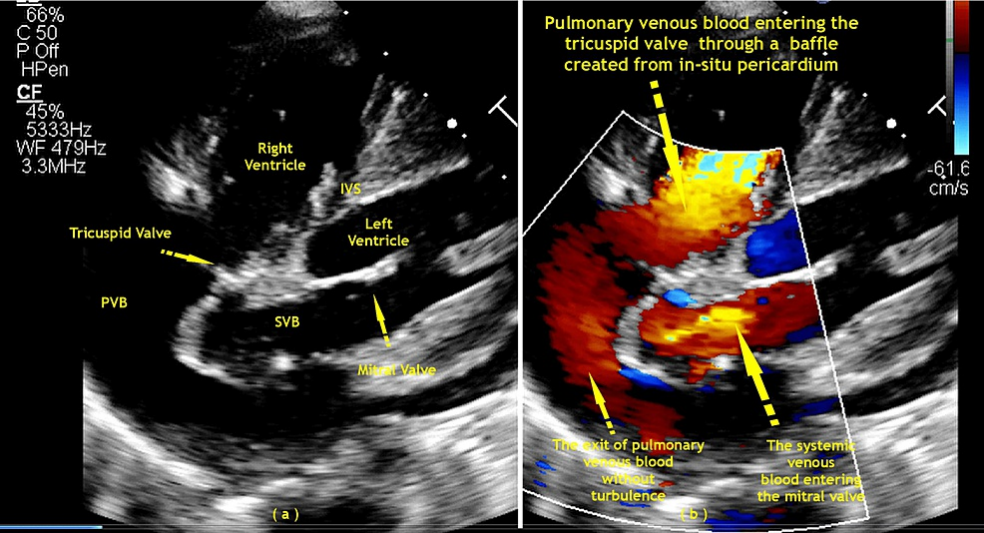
Post-operative 2D echocardiography apical four-chamber images with the posterior tilt of modified Senning’s operation in case 2. (a) 2D echo and (b) color Doppler. PVB: pulmonary venous baffle, SVB: systemic venous baffle, IVS: inter-ventricular septum. Image Credits: Dr. K. S. Ganapathy.
